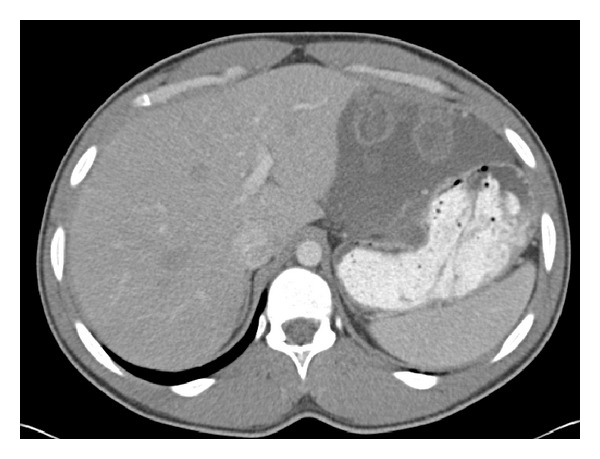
Computed tomography (CT) image demonstrating the appearance of gastric GIST after seven months of treatment with imatinib 400 mg per day; the tumor is unchanged in size compared to initial presentation (initial CT not shown).
